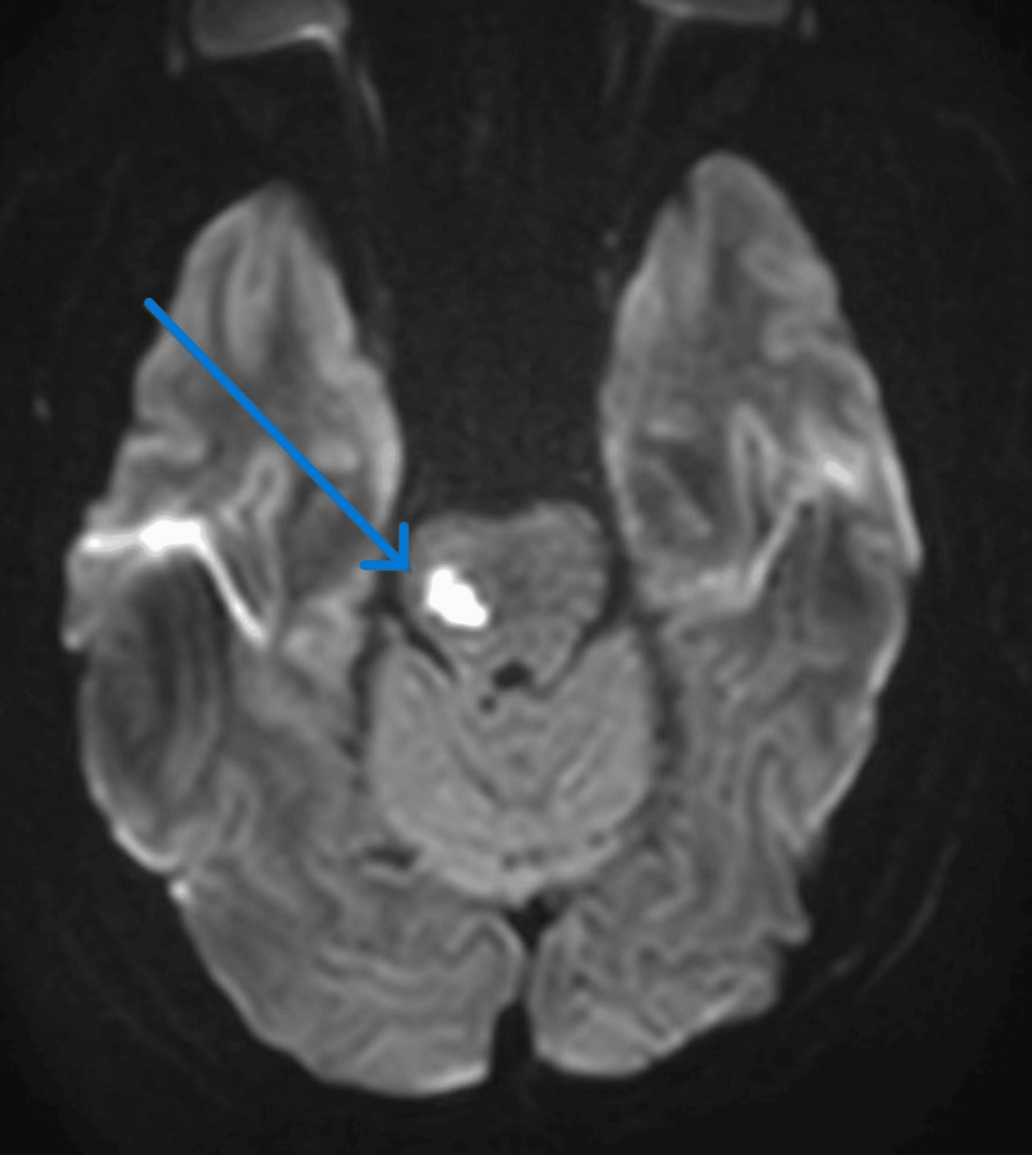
Head MRI shows multiple recent infarcts in the right midbrain (blue arrow).
